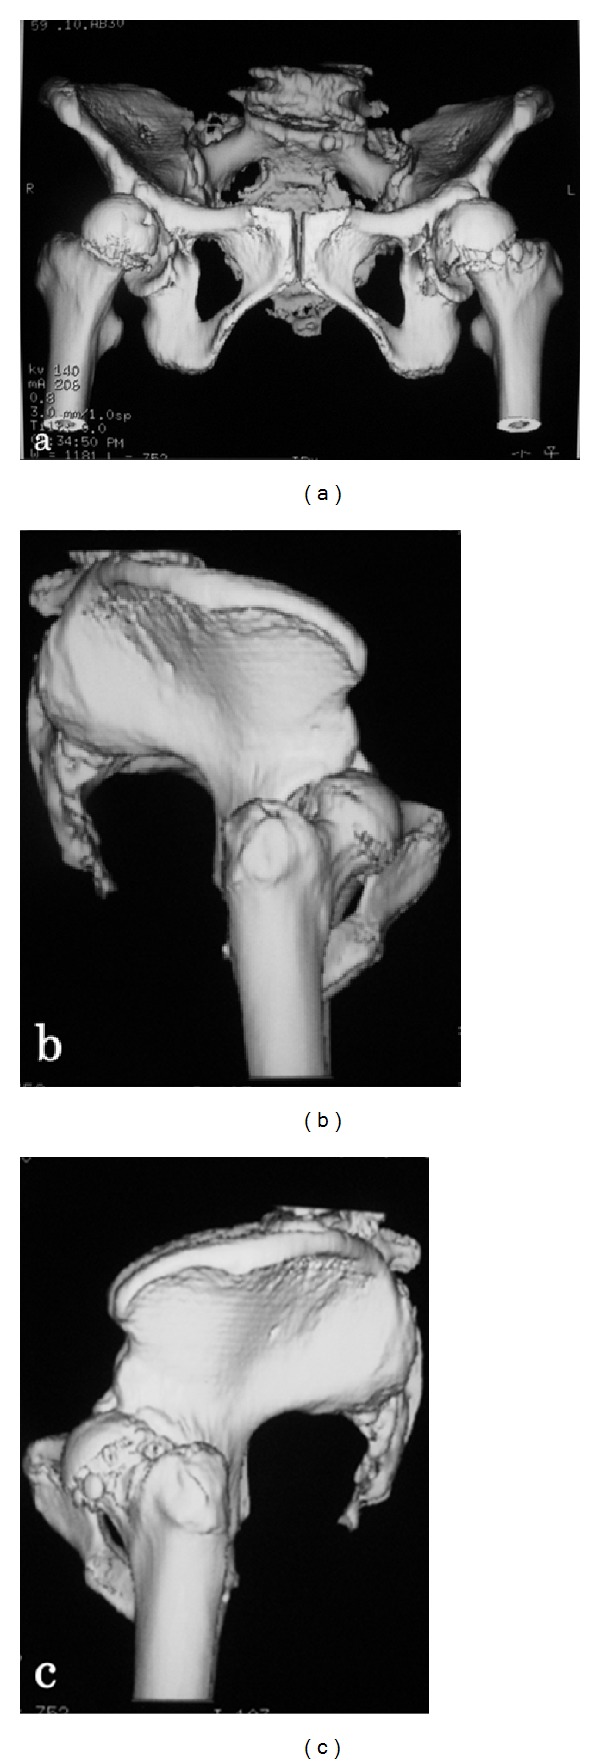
Three-dimensional computed tomography (3DCT) with an anteroposterior view (a) and lateral views of the right (b) and left (c) sides of the bilateral hip joint. 3DCT confirmed anterior subluxation of both femoral heads.
